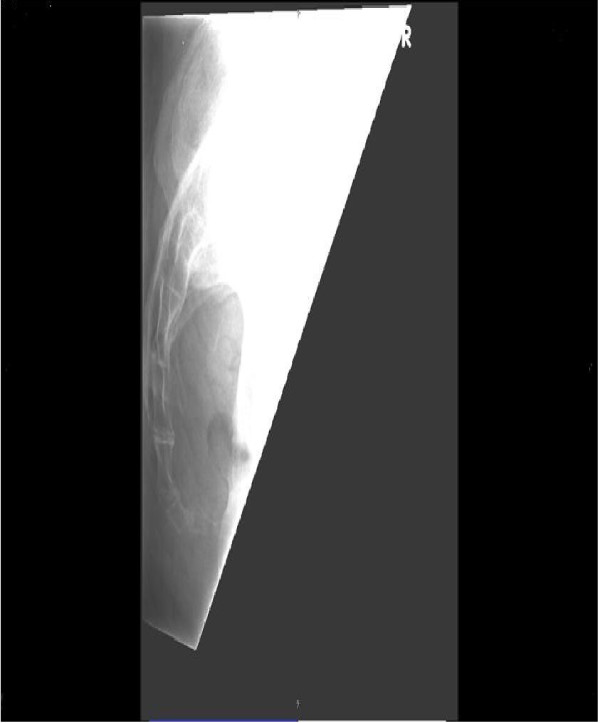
Lateral view X-ray showing an anteverted coccyx.
